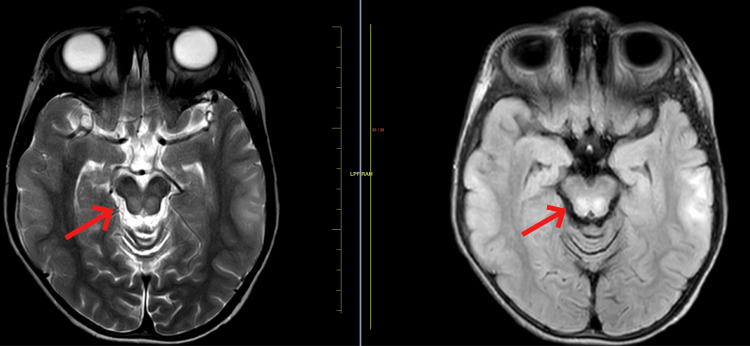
MRI showing T2/FLAIR hyperintense focal lesions noted in brain stem including tegmentum of the midbrain. MRI: Magnetic resonance imaging; FLAIR: fluid-attenuated inversion recovery
